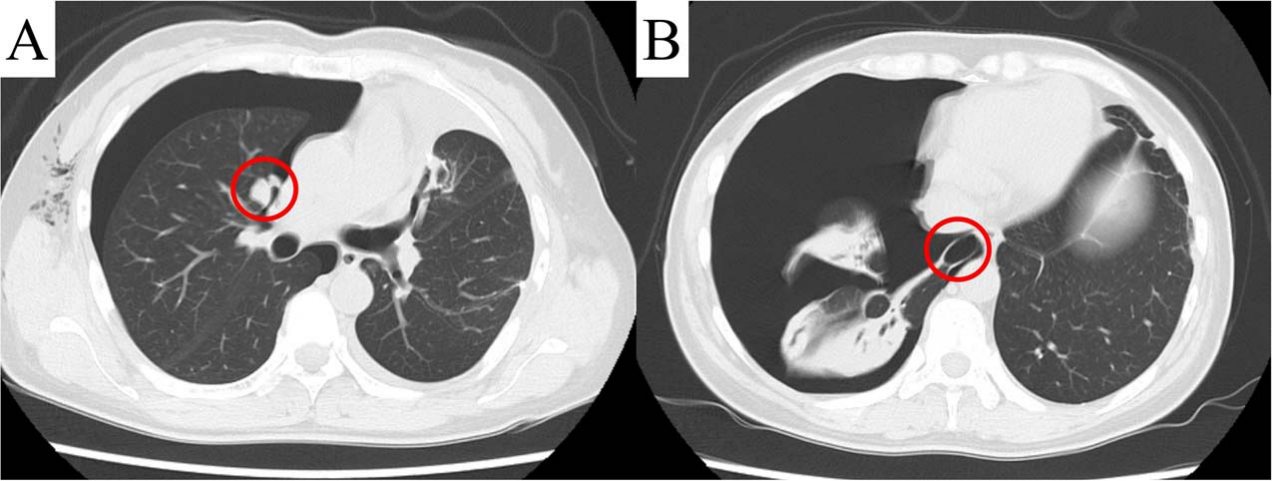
Computed tomography revealed a pleural dissemination nodule in the right hilar area (A) and a bulla in the right lower lobe (B). The findings are indicated by circles.
